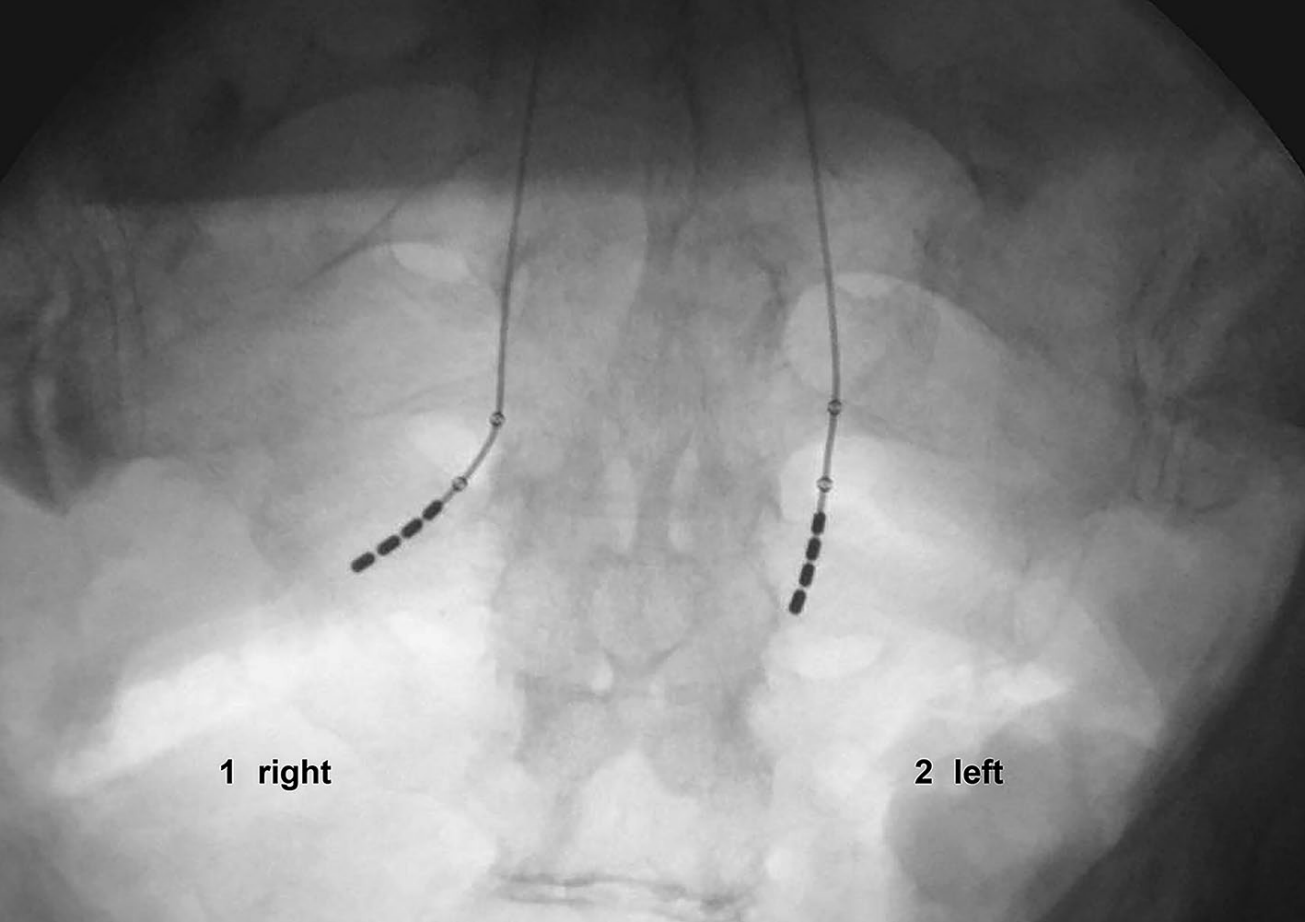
X-ray imaging, anterior–posterior view (1) right side with lateral flexure, (2) left side with straight electrode positioning
